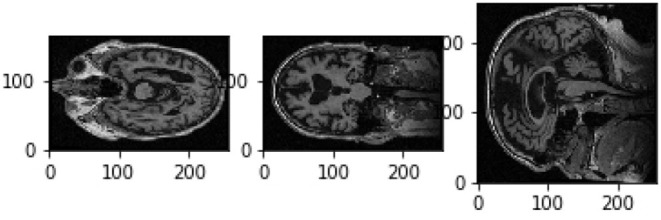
Three slices of a 3D MR image; with sagittal, coronal, and axial plane.
